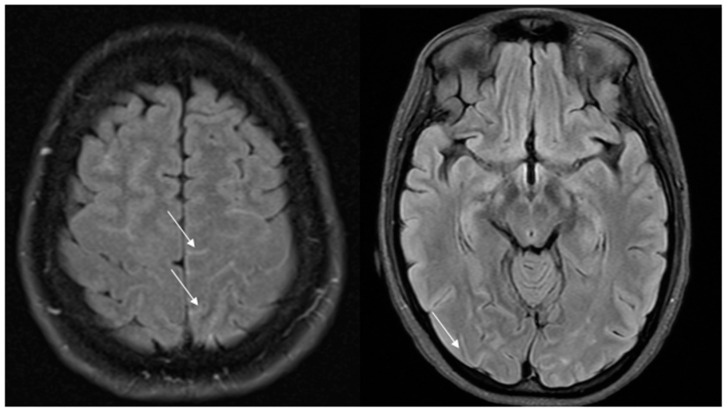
Hyperintense vessels on FLAIR images in two patients with RCVS (arrows).
